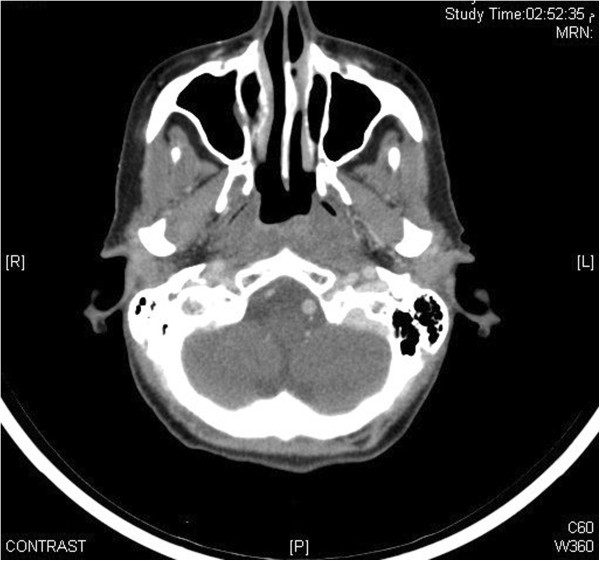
CT head and neck showing recurrent left nasopharyngeal wall mass with some localized contrast enhancement and bulging of mucosal contour without any obvious cervical lymphadenopathy.
